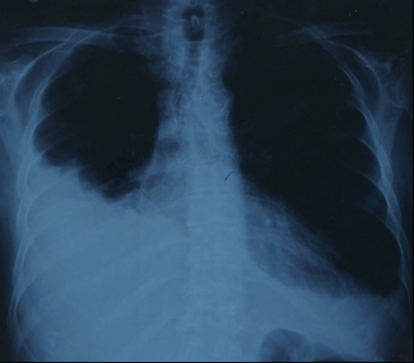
Chest X-ray shows bilateral pleural effusion.
